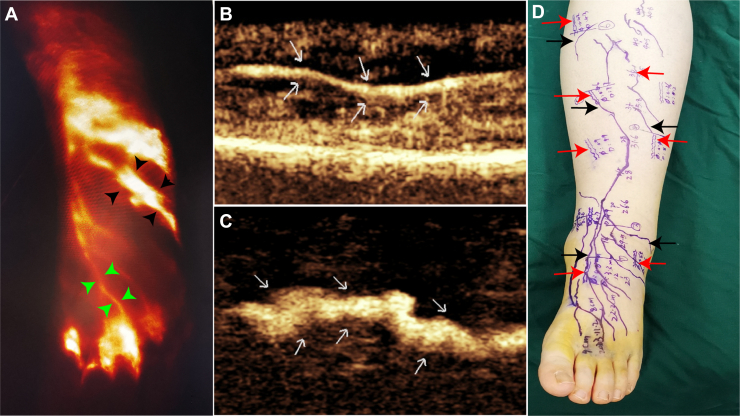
Localization of lower-limb lymphatic vessels in a stage II secondary-lymphedema patient using indocyanine green (ICG) and contrast-enhanced ultrasound (CEUS) before lymphovenous anastomosis (LVA) surgery. (A) ICG image of the dorsum of the foot showing lymphatic vessels (*green and black short arrows*). The *green arrow* indicates a lymphatic vessel with normal diameter and course, whereas the *black arrow* indicates a dilated lymphatic vessel. (B) CEUS image at the *green arrow* site in panel (A) showing a lymphatic vessel with normal course and no dilation (*white arrow*). (C) CEUS image at the *black arrow* site in panel (A) showing a tortuous, dilated lymphatic vessel. (D) Surface markings of the lymphatic vessels: *black arrows* indicate ICG-based markings, and *red arrows* indicate CEUS-based markings.
